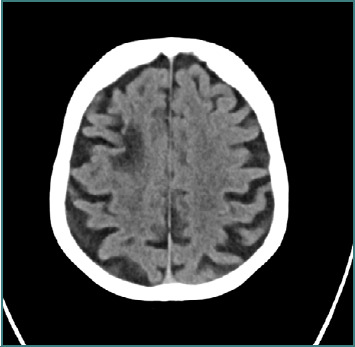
Non-contrast brain CT showed an encephalomalacia and gliotic scar in the right frontal lobe, consistent with prior ischemic injury
